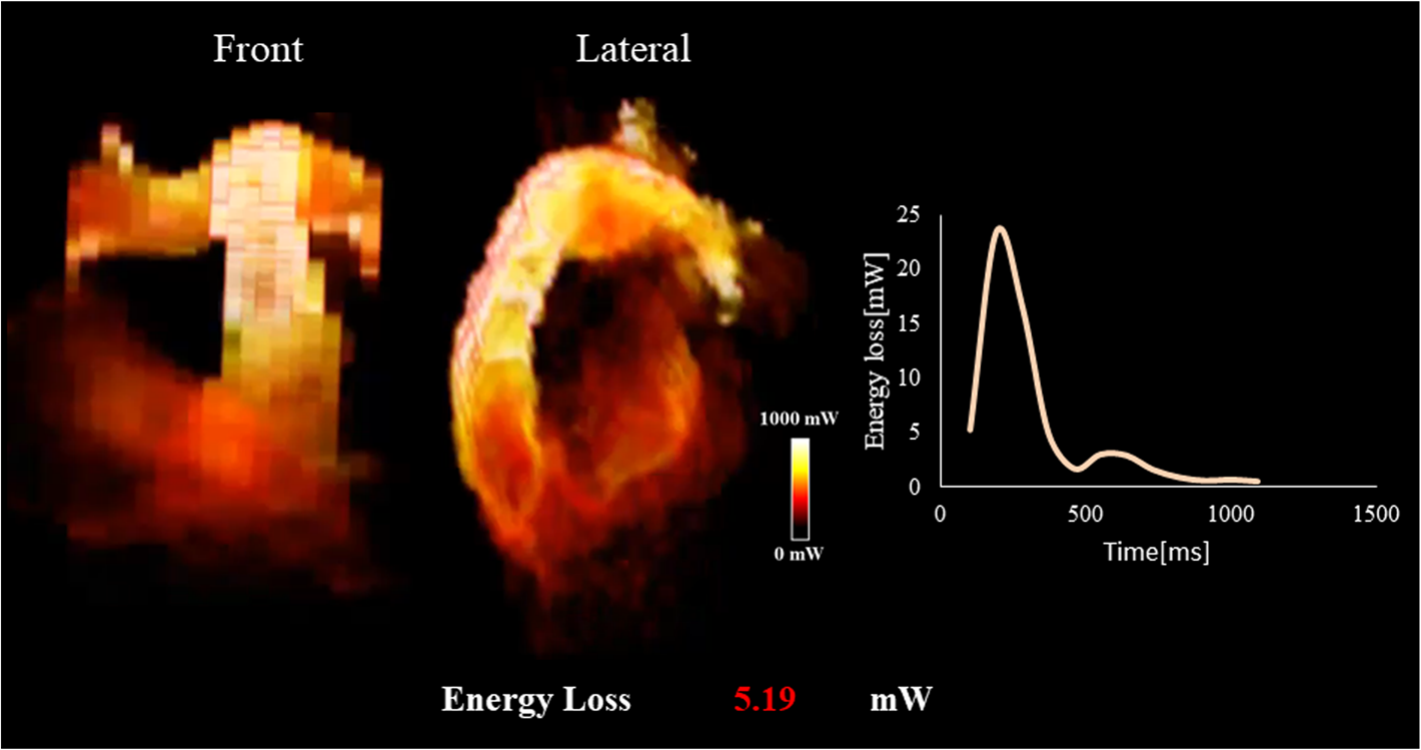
Flow energy loss. Flow energy loss in right ventricle calculated from four-dimensional flow magnetic resonance imaging was 5.19 mW, which is estimated to be five times higher than normal controls
